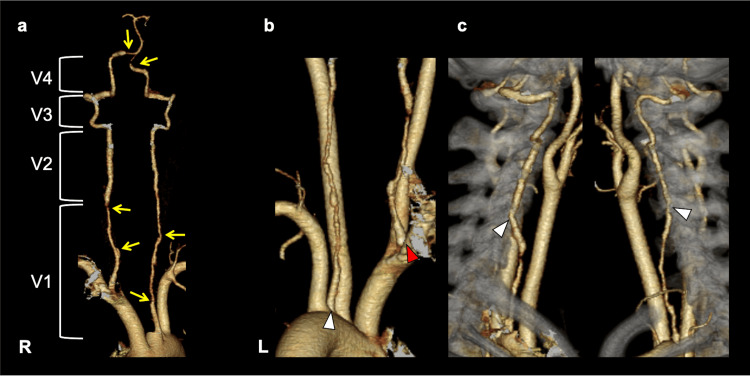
Head and neck CT angiogram (CTA) a. Irregular caliber of the bilateral V1 segments: broad, and portions of the bilateral V4 segments (yellow arrows); b. The left vertebral artery originated directly from the aortic arch (white triangle), and the right vertebral artery originated immediately after the branching of the brachiocephalic trunk (red triangle); c. Both vertebral arteries showed an anomalous high transverse foramen (C4) penetration (white triangles).
